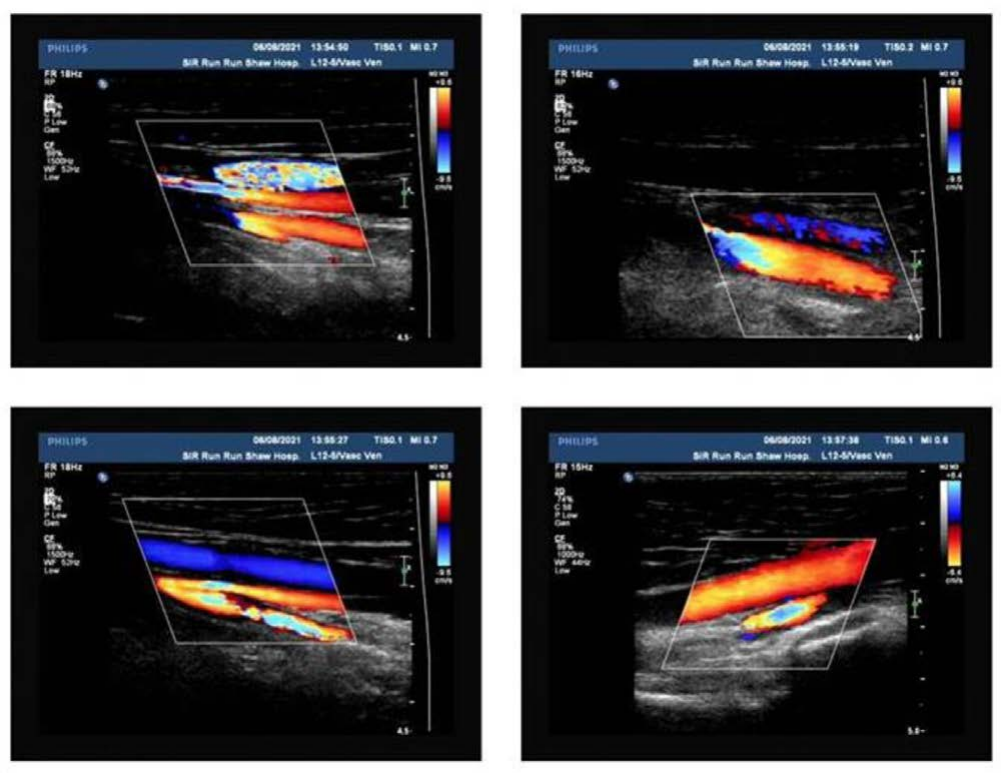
Dual-function ultrasound examination of the veins confirmed that the blood flow of the deep veins of both lower limbs was unobstructed.
